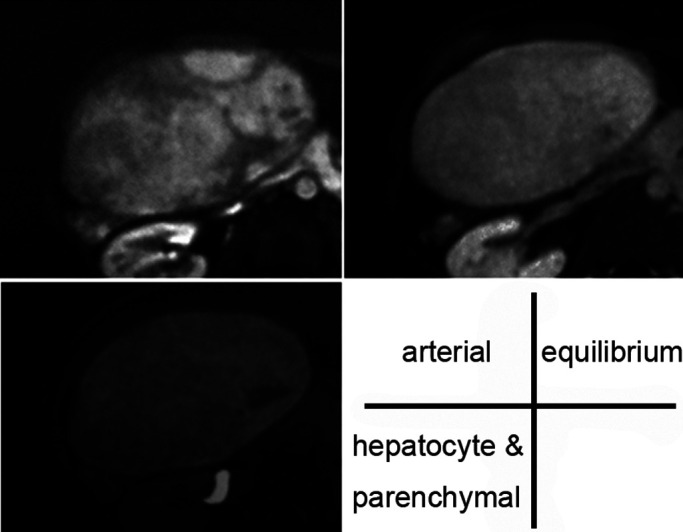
Contrast MRI With a non-uniform enhancement effect after contrast in EOB, washout in the equilibrium
